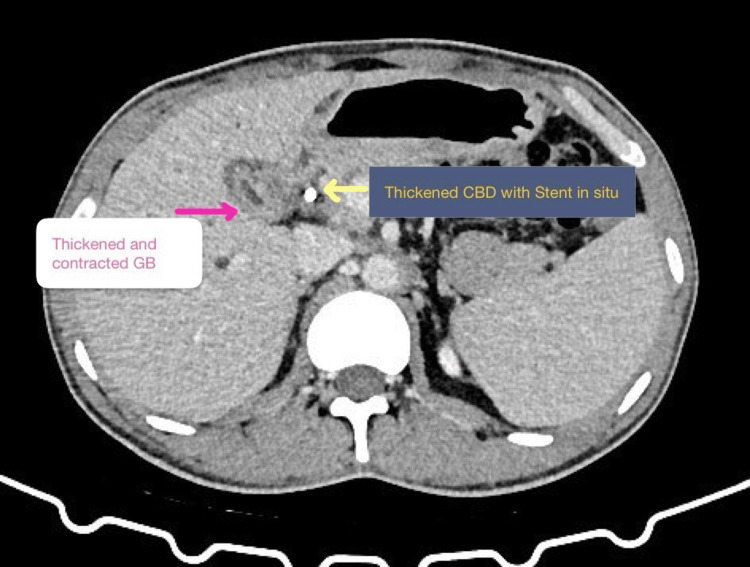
CT scan-thickened GB neck and CBD with a stent in situ. Axial section of CT scan. GB: gall bladder; CBD: common bile duct.
